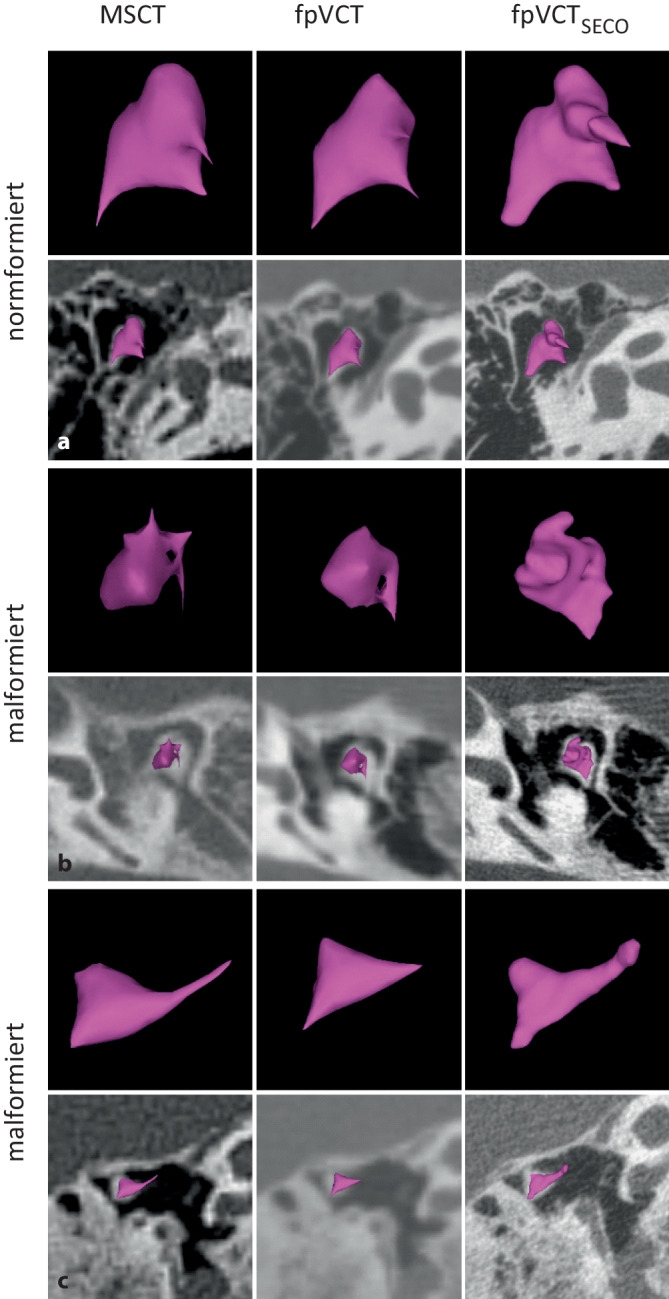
3‑D-Darstellung des Malleus-Incus-Komplexes in der Planungssoftware OTOPLAN® mittels MSCT (600 µm Schichtdicke), fpVCT (466 µm Schichtdicke) und fpVCT_SECO_ (99 µm Schichtdicke). Die Komplexe sind jeweils isoliert und mit einer überlagerten axialen Ebene dargestellt. a zeigt einen normal konfigurierten Komplex. Ein mäßig fehlgebildeter Komplex ist in b und ein stark fehlgebildeter Komplex in c dargestellt. *MSCT* Multislice-Computertomographie, *fpVCT* „flat-panel volume computed tomography“, *fpVCT*_*SECO*_ sekundäre Rekonstruktionen der fpVCT
